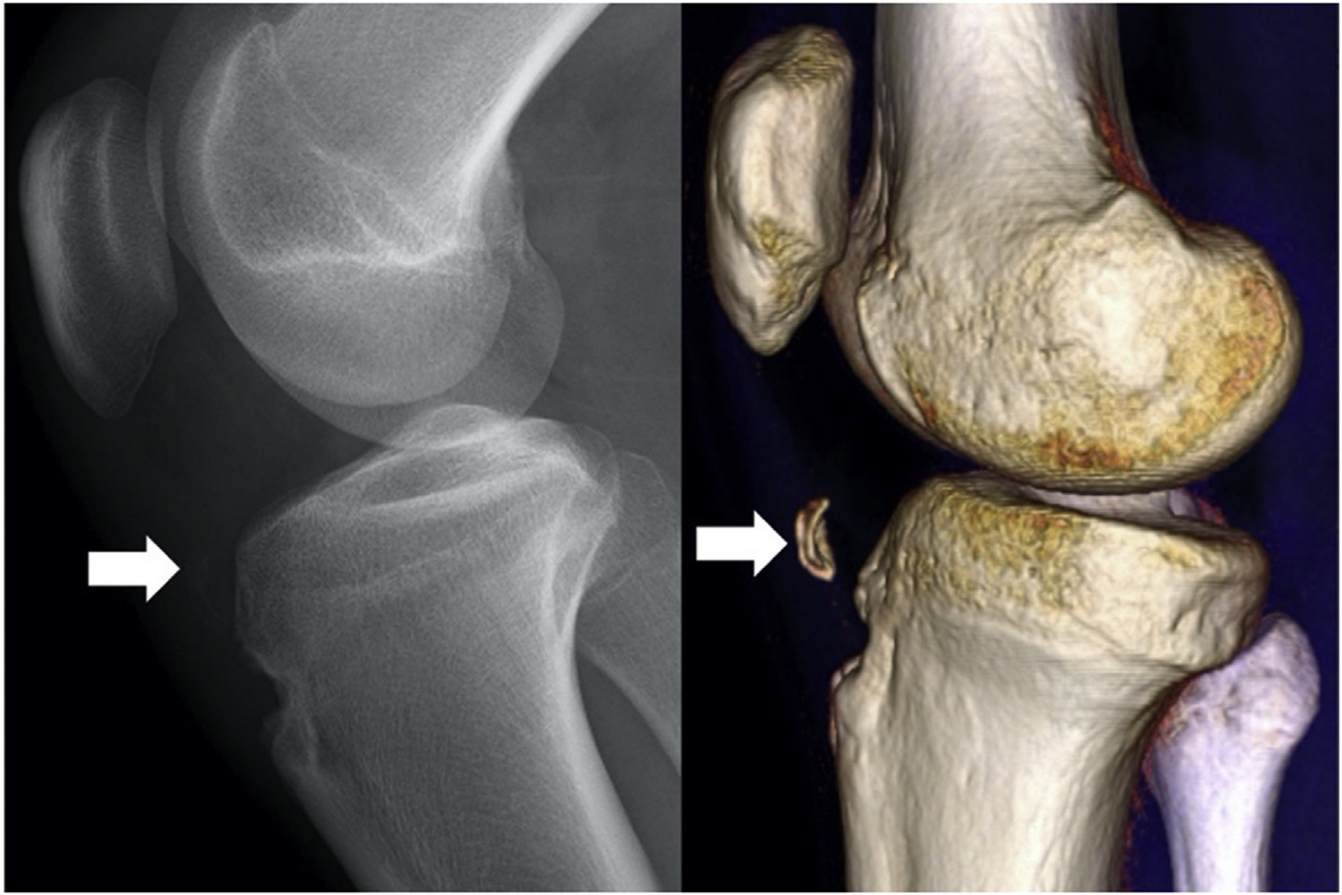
View on lateral radiograph (A) and 3-dimensional CT scan (B). The ossicle is shown (arrow). The ossicle may be difficult to identify plain radiographic images.
